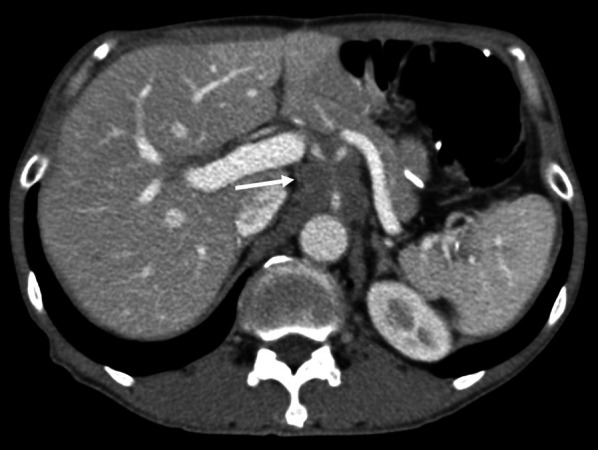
Computed tomography revealed a swollen lymph node (35 mm × 33 mm) in station no. 9 along the celiac artery (arrow)
